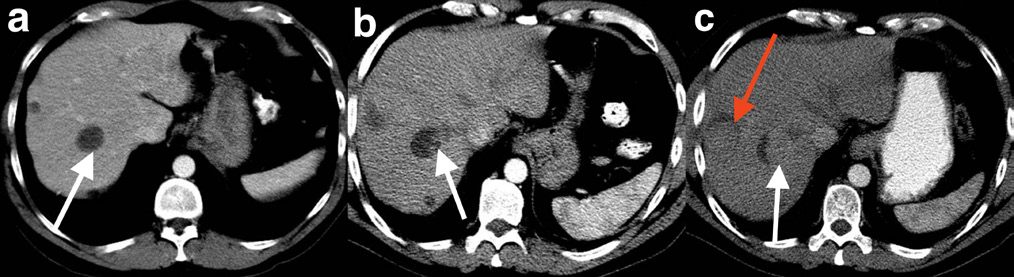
Recurrent liver metastases. Portal venous CT shows treated cystic attenuation of GIST liver metastases - the largest is located in segment 7 (a). CT performed 3 months later demonstrates subtle nodularity within the largest lesion (b). This was not recognised. A study 3 months later shows increase in size of the nodule within the cystic metastasis (white arrow) as well as recurrence of a smaller peripheral segment 7 lesion only partially imaged (red arrow) (c). GIST, gastrointestinal stromal tumour
